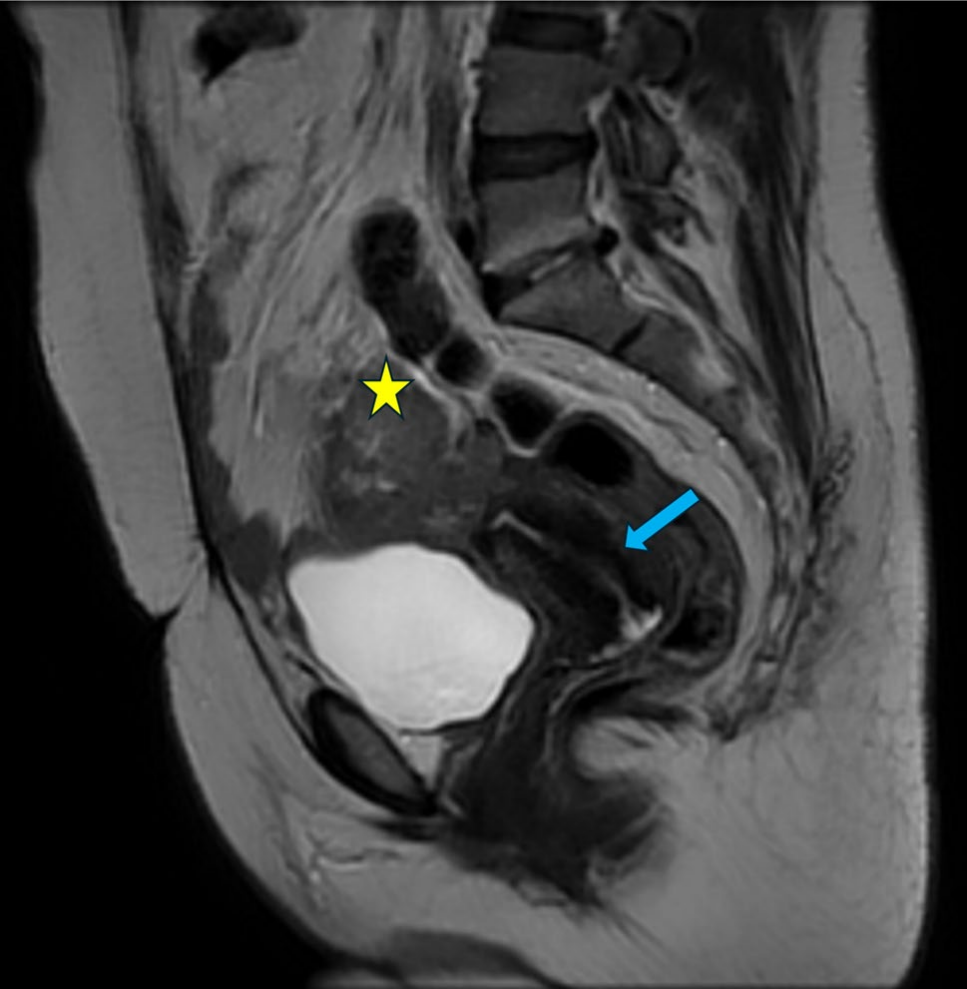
Sagittal T2W image showing the remaining cervical stump with its endometrial lining (blue arrow) and a heterogeneous solid lesion (yellow star) above the cervix with its MRI signal matching with fibroid
